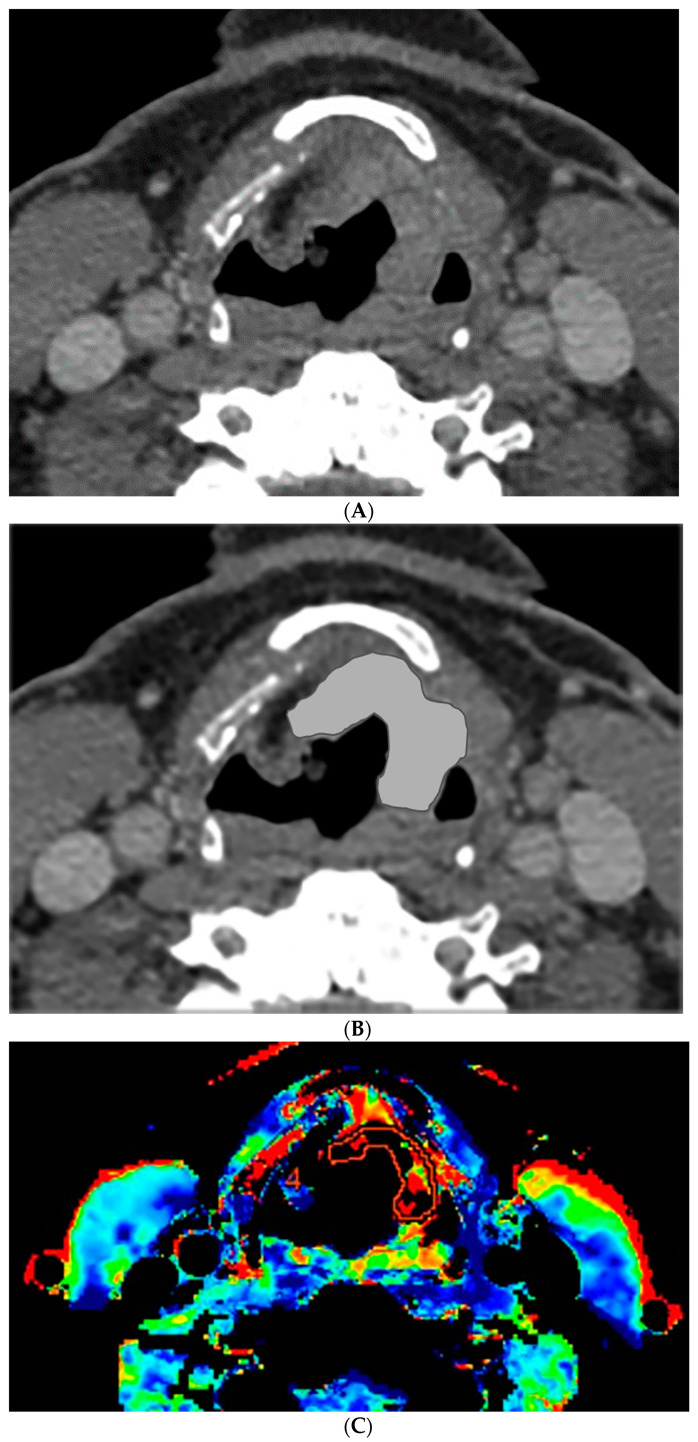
CT and perfusion sections of laryngeal carcinoma on post-treatment images. Axial sections show the grayscale image (A) and outlines for the following: computer (B) and perfusion (C).
